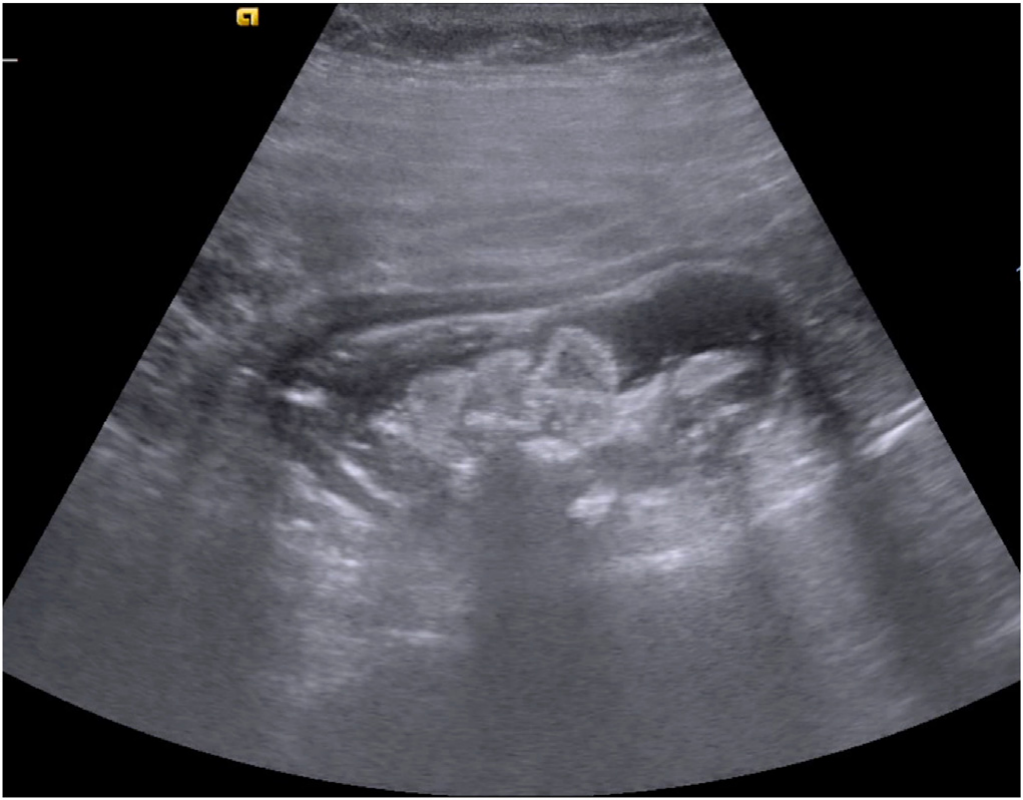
Intestinal ultrasound demonstrating an ascending colon stricture with upstream fecaliths, confirming luminal narrowing and prestenotic dilation.
